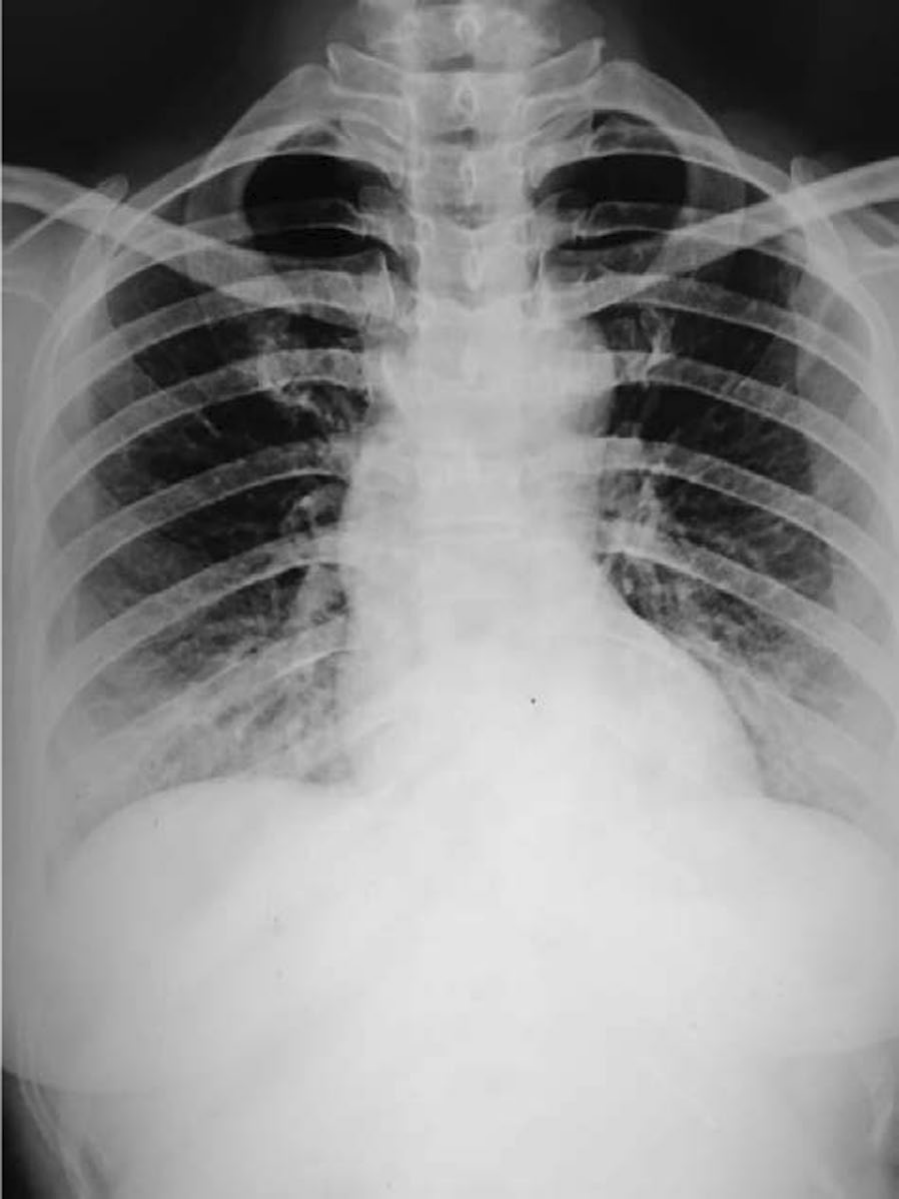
Chest X-ray showing prominent interstitial markings
